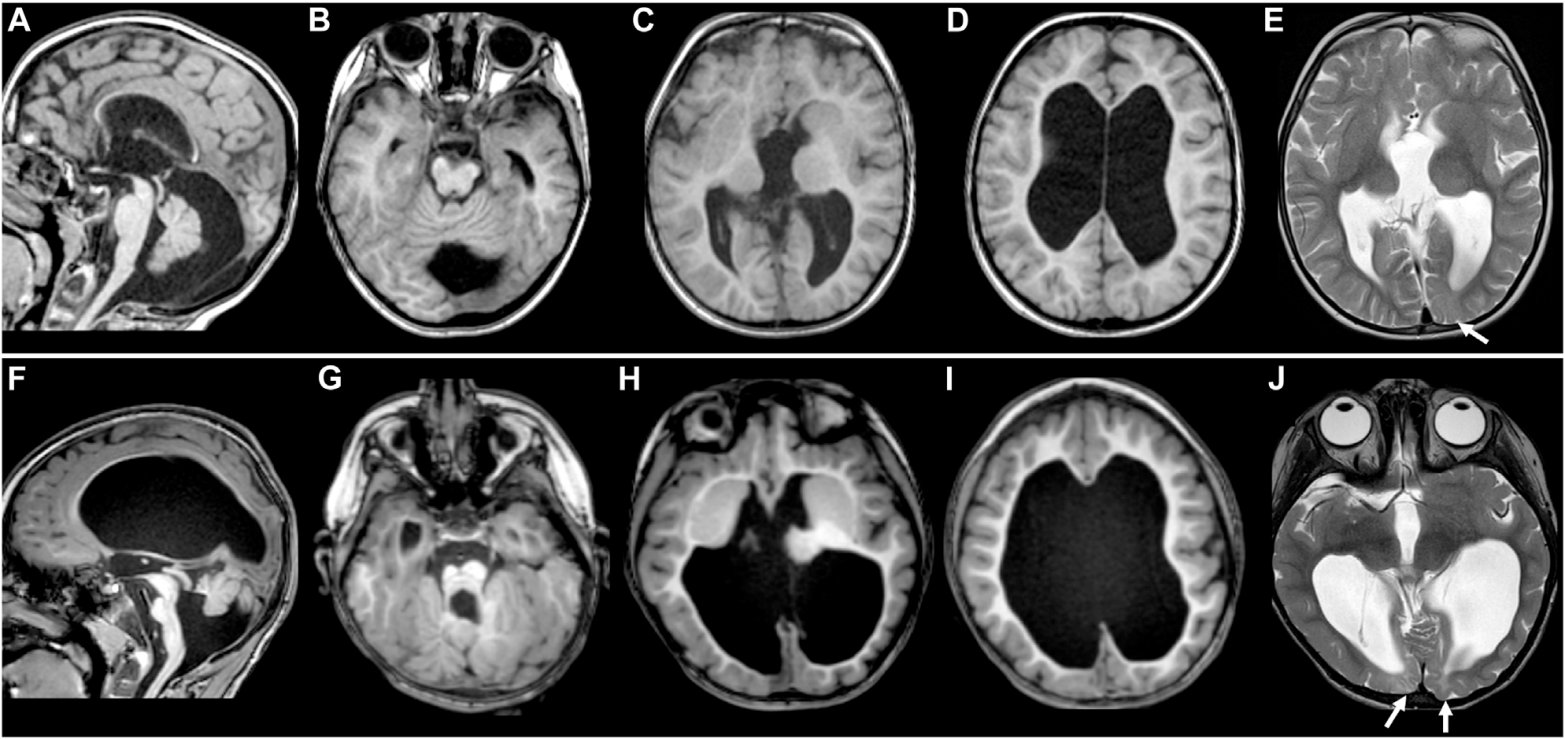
MR images of *TUBB2A*-T178M and *TUBB3*-T178M patients. Selected axial T1 (A–D) and T2 (E) MR images of patient #1 (with *TUBB2A* mutation) obtained at age 4 demonstrate common features of a moderate tubulinopathy MR phenotype, including (A) mildly small pons and vermis, thin corpus callosum; (B) small, asymmetric-appearing brainstem, mild superior vermian disorganization; (C) abnormal, asymmetric basal ganglia with enlarged caudate and thin anterior limb internal capsule separating the caudate and putamen; (C,D) diffuse pattern of tubulinopathy-associated dysgyria consisting of normal-thickness but closely spaced and disorganized-appearing sulci with orientation directed radially to a central point; (D) ventriculomegaly with low white matter volume. (E) also demonstrates asymmetric T2 hyperintensity in the juxtacortical white matter of the left occipital lobe (arrow), with preservation of overlying cortex. Selected axial T1 (F–I and T2 (J) MR images of patient #2 (with *TUBB3* mutation) obtained at age 3 demonstrate similar features as patient #1, but of a more severe MRI phenotype, including (F) moderately to markedly small pons and vermis with posterior vermian rotation, mildly thickened tectum relative to brainstem size, thin corpus callosum; (G) small, asymmetric-appearing brainstem, moderate/severe superior vermian and cerebellar foliar disorganization; (H) abnormal, asymmetric basal ganglia with no discernable separation of the caudate head and putamen; (H,I) tubulinopathy-associated dysgyria with shallow, closely spaced and disorganized-appearing sulcation also directed radially to a central point; (I) ventriculomegaly with low white matter volume. (J) shows more extensive and intense abnormal T2 hyperintensity in the juxtacortical white matter of the bilateral occipital lobes (arrowheads), with preservation of overlying cortex.
